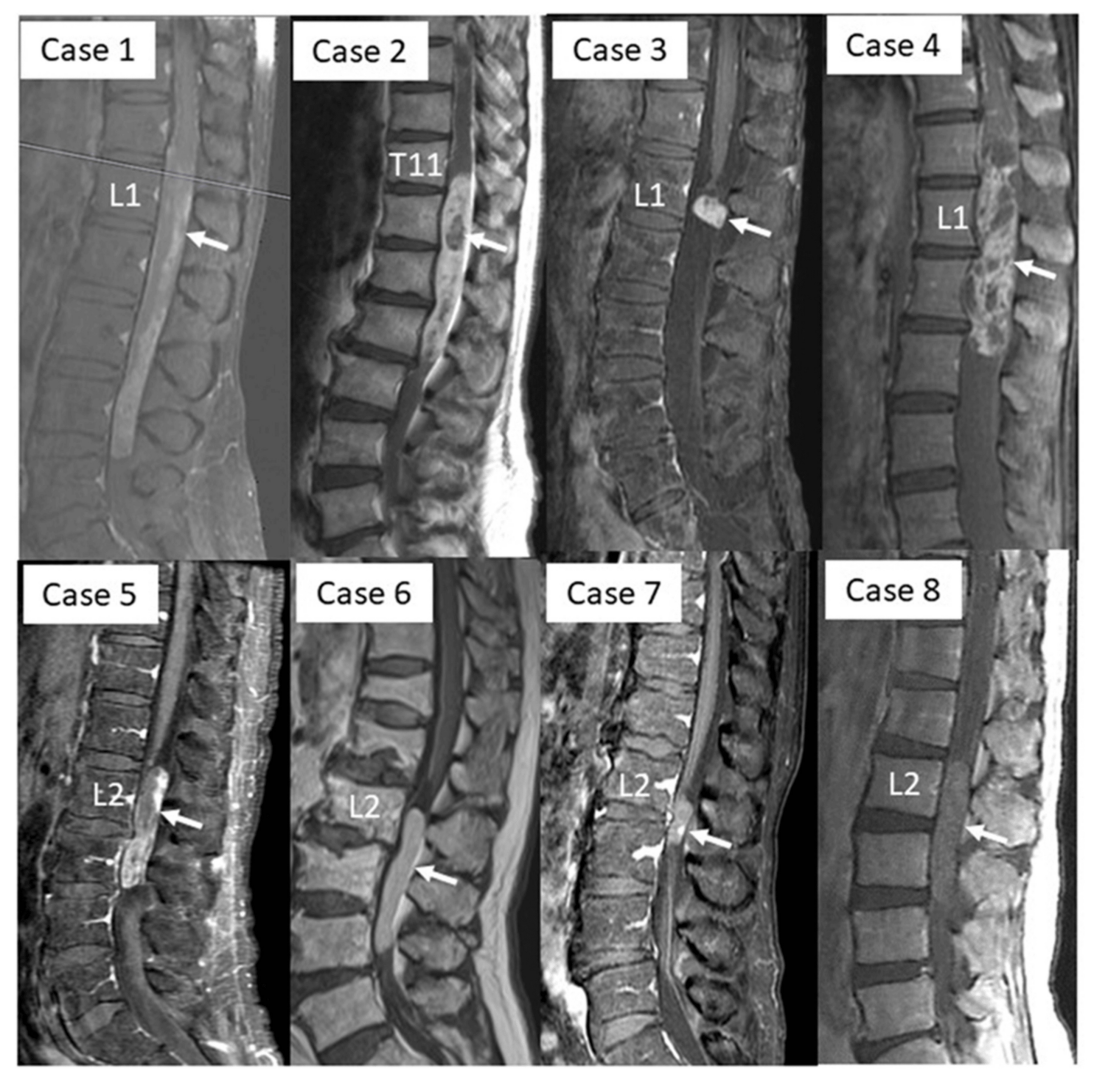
Preoperative enhanced MRI. White arrows indicate the cranial side of tumor location.
